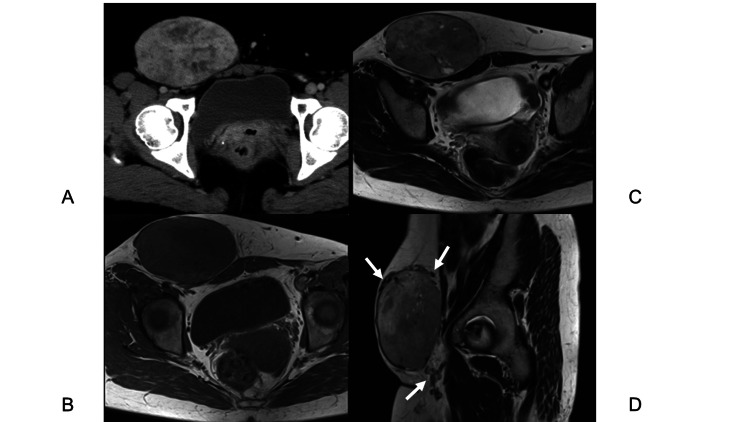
Contrast-enhanced CT and MRI of the pelvis (Case 2) A well-defined 11.8×12.2×6.9 cm tumor covered by a capsule and showing heterogeneous enhancement on contrast-enhanced CT (A: axial view), low- to iso-intensity on T1-weighted imaging (B: axial view) and heterogeneous intensity on T2-weighted imaging (C: axial view) is observed within the subcutaneous fat tissue adjacent to the rectus abdominis surface. Tortuous vascular structures (arrows) are proliferating around the tumor on T2-weighted imaging (D: sagittal view).
